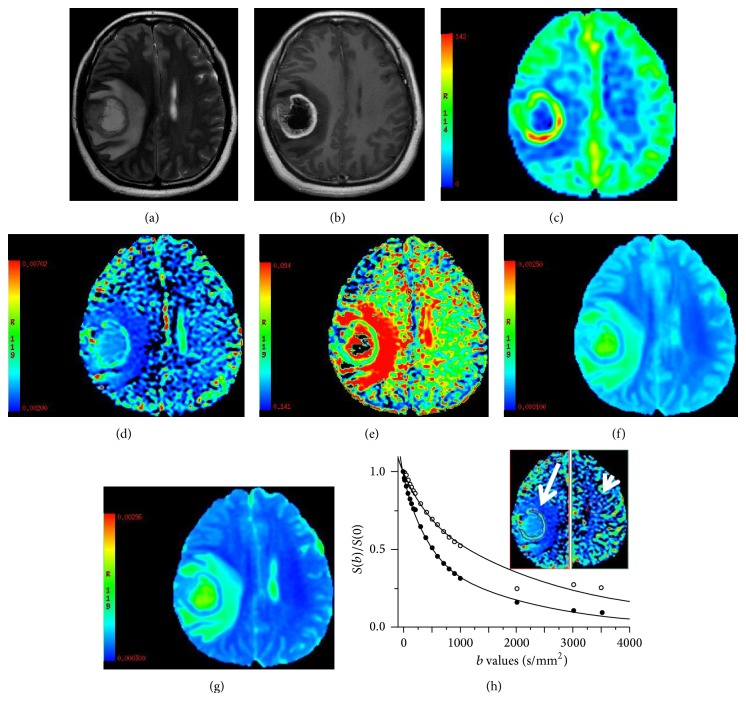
Female, aged 43 years with a glioblastoma of the right parietal lobe (WHO IV). The axial T2-weighted image (a) shows the medium signal thick walled mass with a hyperintense central cystic necrosis and peritumoral edema. Enhanced T1-weighted images (b) show the significantly enhanced tumor wall. CBF map (c) shows that the wall of the mass with unevenly high perfusion; the TBF and CBF of contralateral semioval center were 89.90 and 16.89 mL/100 g/min, respectively. The *D*
